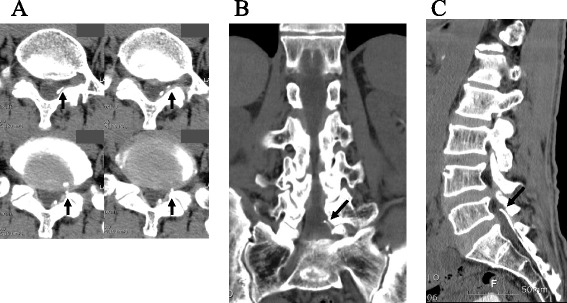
Lumbar spine three-dimensional computed tomography before catheter fragment removal. a Computed tomography axial view showing the compression of the left lumbar vertebral body 5 nerve root by a broken catheter tip (*arrow*). b Computed tomography coronal view showing that the catheter fragment tip is located at the left side of the lumbar vertebral body 5/sacral vertebral body 1 foraminal zone (*arrow*). c Computed tomography sagittal view showing the catheter fragment tip which spans from the sacral hiatus to the lumbar vertebral body 5/sacral vertebral body 1 level (*arrow*)
